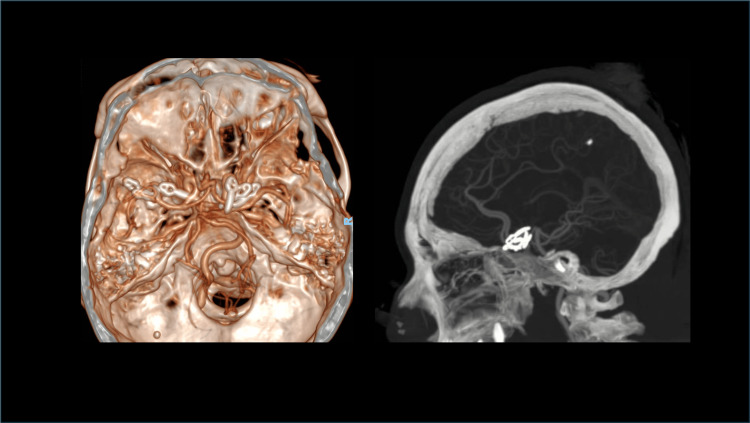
Three-dimensional reconstruction from postoperative CT angiography Postoperative imaging demonstrating sequential proximal-to-distal clipping of three aneurysms, followed by definitive clipping of the blister aneurysm. This stepwise strategy provided an unobstructed operative field and facilitated safe and accurate clip placement.
